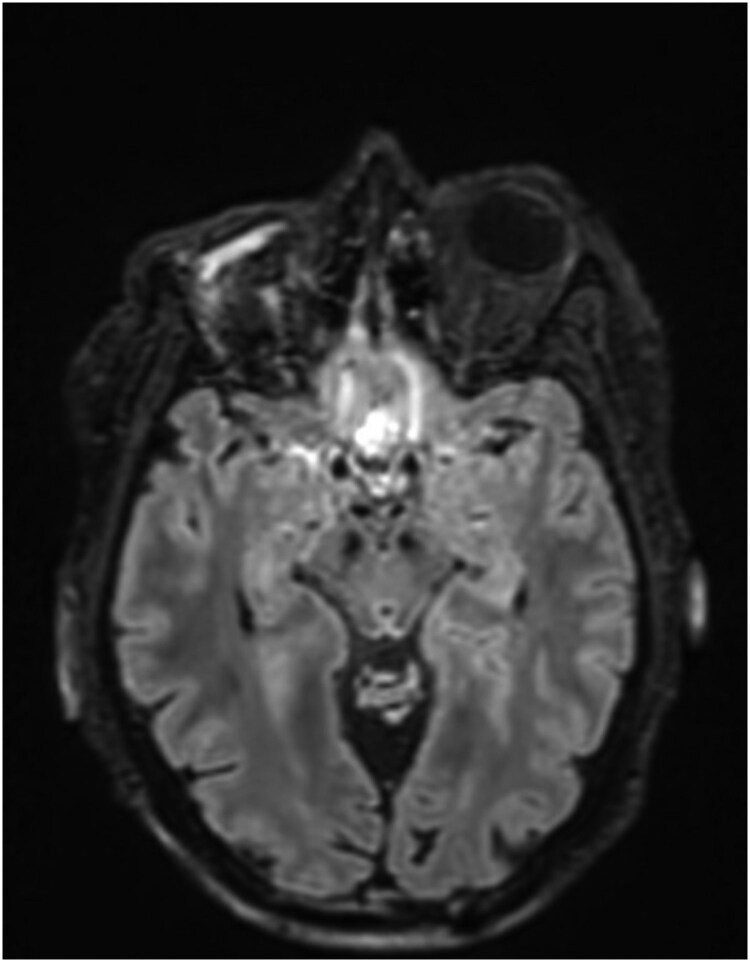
Magnetic resonance imaging of the brain showing postsurgical changes of the right orbital excision are with enhancing soft tissue mass involving the preorbital soft tissues and the floor and right lateral wall of right anterior cranial fossa.
